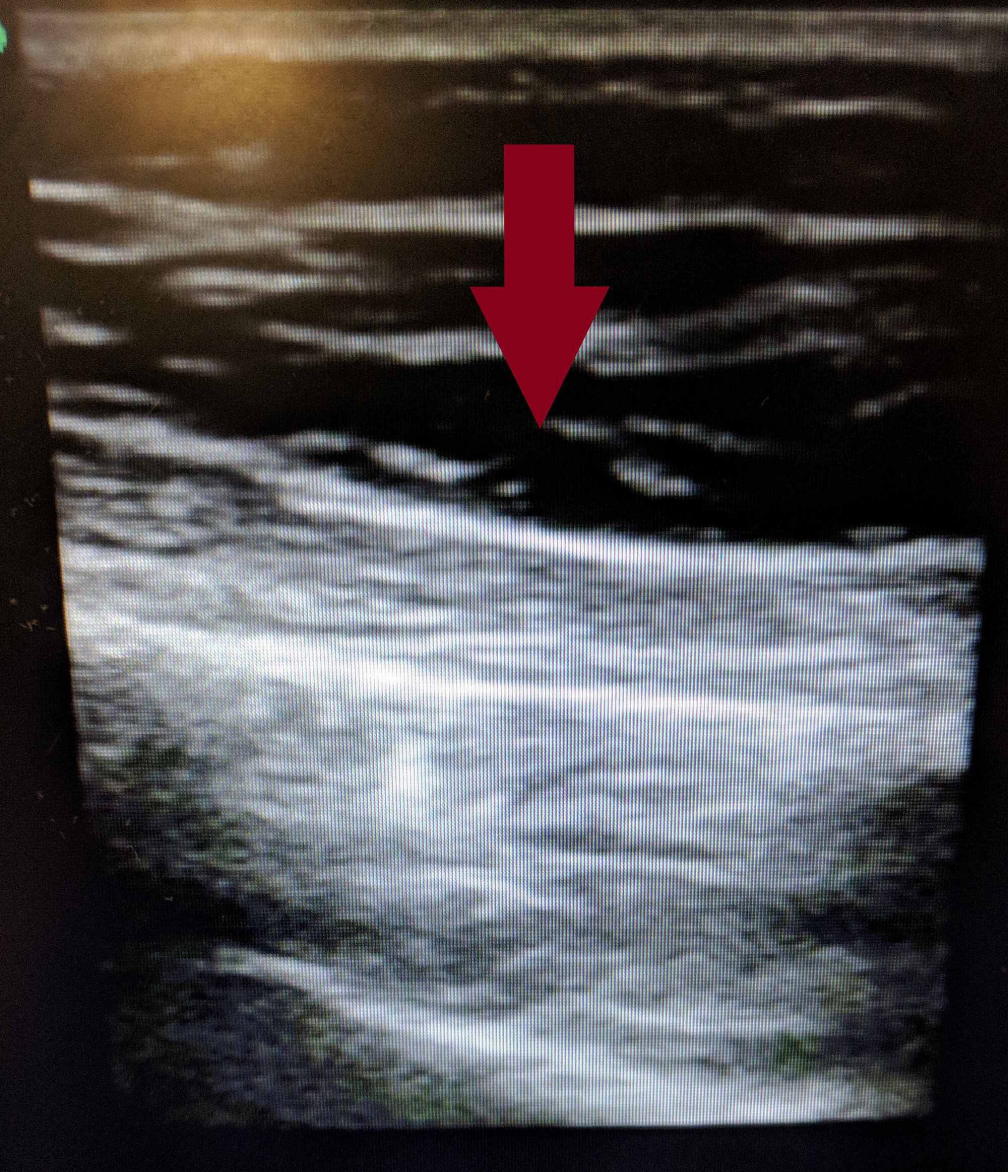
Bedside ultrasound revealing fluid in the fascial planes of the right chest wall.
